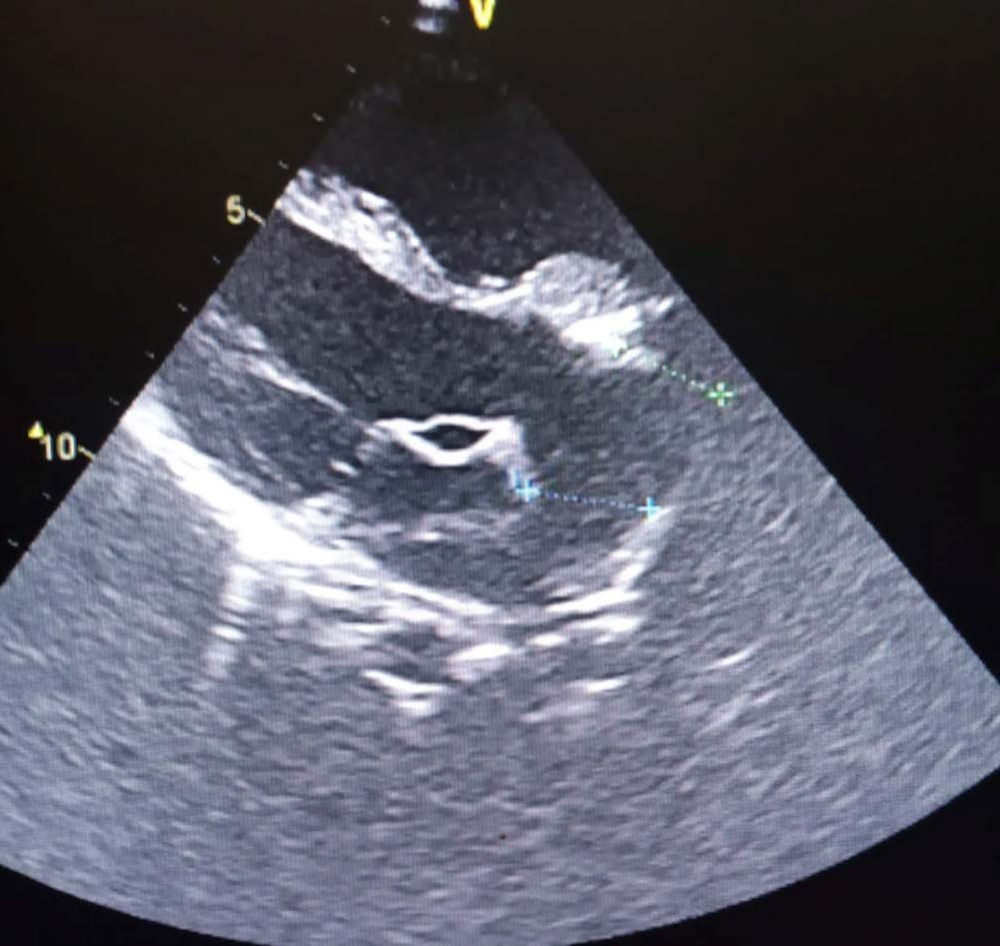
Echocardiographic image showing the aorta arising from the left ventricle and bifurcating into left and right pulmonary trunk.
